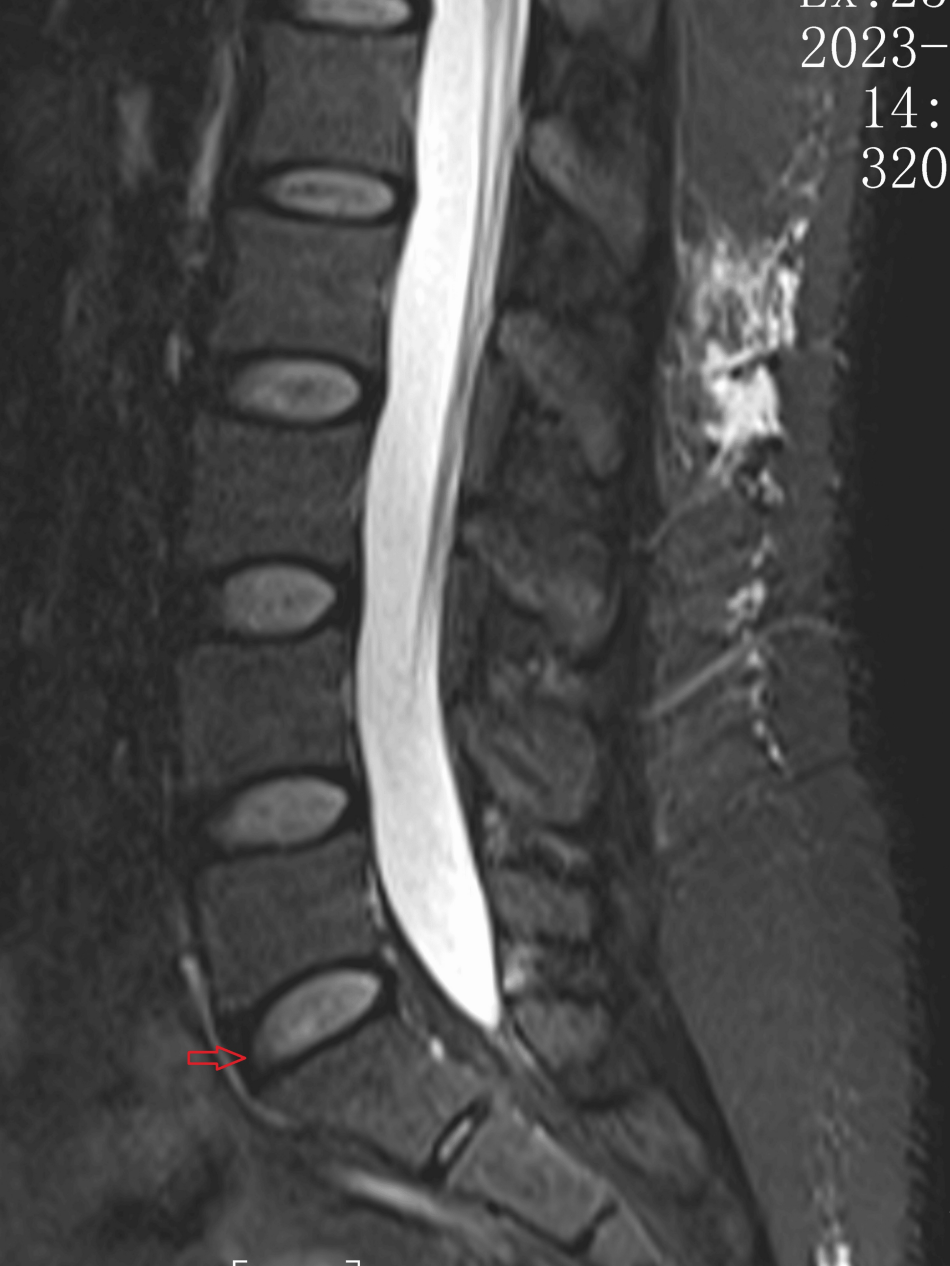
MRI of the lumbar spine The red arrow points to the lumbar intervertebral disc. T2W-FS shows no disc herniation, with posterior fascial edema.
